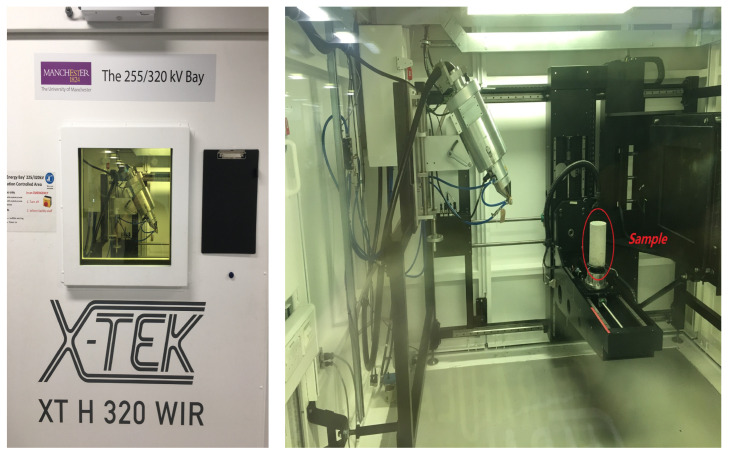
Nikon XTH 225 custom bay in Henry Moseley X-ray Imaging Facility.
